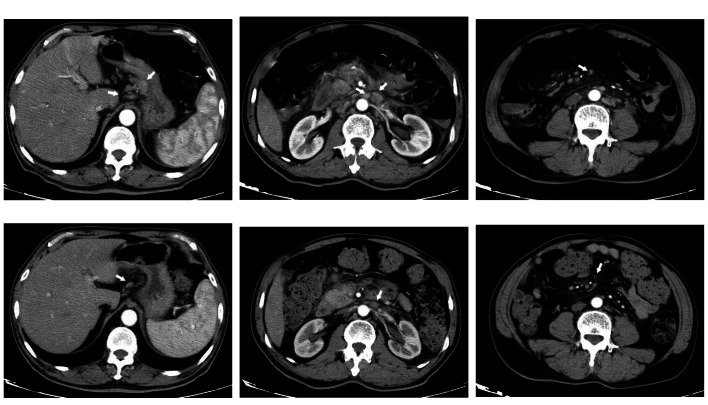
CT examination (A–C) prior to chemotherapy and (D–F) following two cycles of chemotherapy. Arrows show metastatic lymph nodes. CT, computed tomography.
